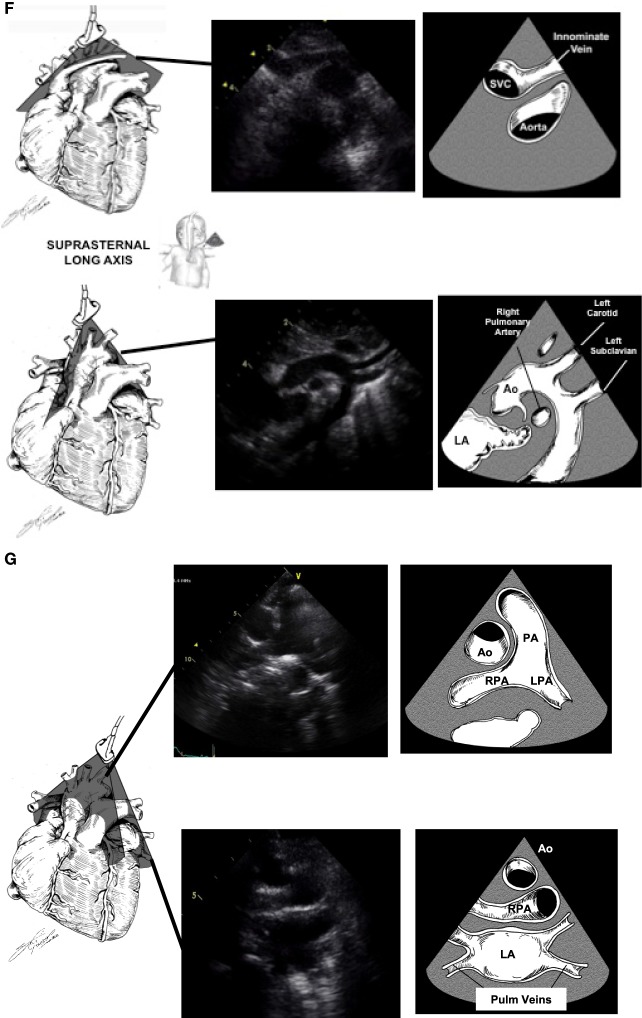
Standard echocardiographic image planes from the high left chest just lateral to the sternum [parasternal window (A,B)], the left lateral chest just inferior and lateral to the nipple [apical window (C)], sub-xyphoid area [subcostal window (D,E)], and the suprasternal notch [suprasternal window (F,G)]. Ao, aortic valve; CS, coronary sinus; LA, left atrium; LV, left ventricle; PA, pulmonary artery; RA, right atrium; RV, right ventricle; RVOT, right ventricular outflow tract; SVC, superior vena cava. Adapted from Ref. (1).
